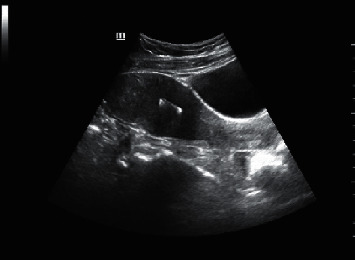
Ultrasound image of uterine fibroids of a female patient who underwent GLS (35 years old).
